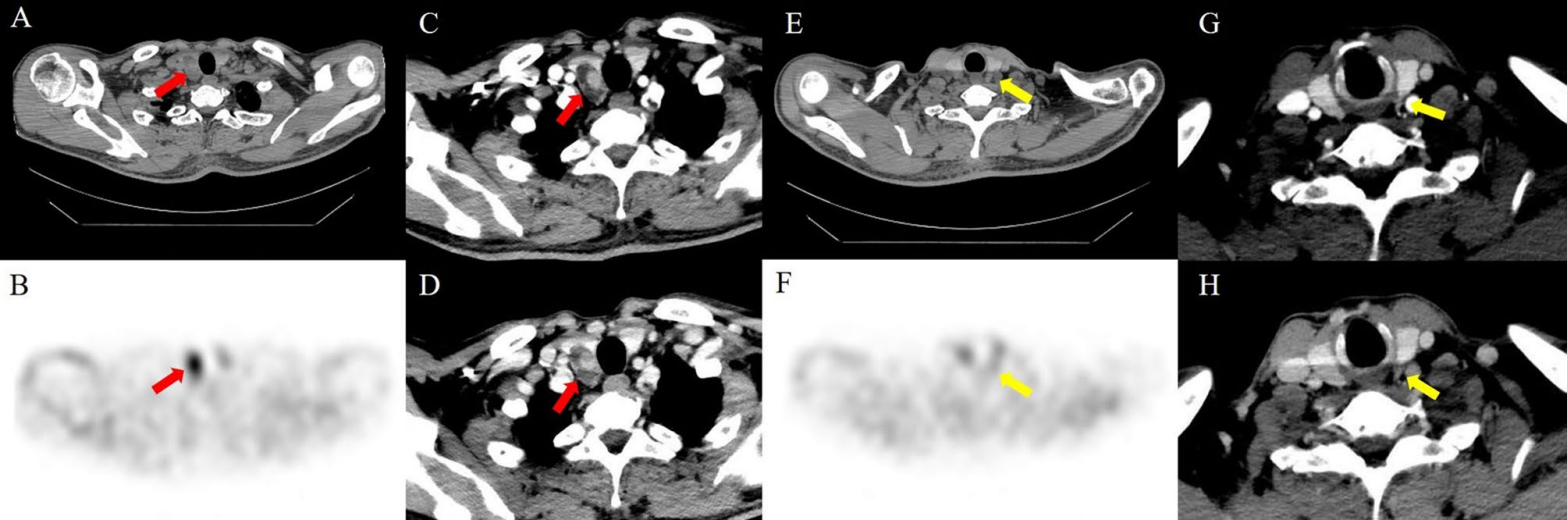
Image of a 57-year-old man with primary hyperparathyroidism. Axial noncontrast-enhanced CT (A) image showing an oval lesion (red arrows) localized to the right inferior parathyroid gland. Axial sestamibi SPECT image (B) showing focal radioactivity uptake localized to a single abnormal right superior parathyroid gland (red arrow). Axial 4D-CT (C, arterial phase; D, delayed phase) reveals an enhanced right inferior parathyroid gland (arrows) corresponding to the same gland identified via sestamibi SPECT/CT. Additional mild focal radioactivity uptake was noted in the left superior parathyroid gland (yellow arrows) on sestamibi SPECT/CT (E, axial noncontrast-enhanced; F, axial SPECT), which was not obscured on 4D-CT (G, arterial phase; H, delayed phase). The patient underwent bilateral four-gland exploration for left superior parathyroid hyperplasia and right inferior parathyroid adenoma. Normal PTH and calcium levels were observed after 19 months of follow-up
